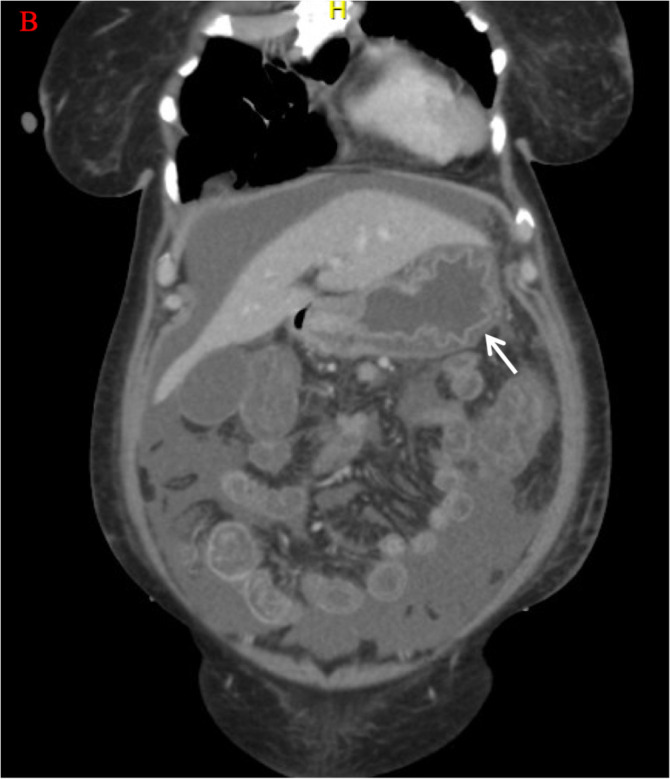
CT Angiogram abdomen: circumferential, symmetrical, multisegmented mural thickening with associated submucosal oedema (Target sign) seen (arrows).
